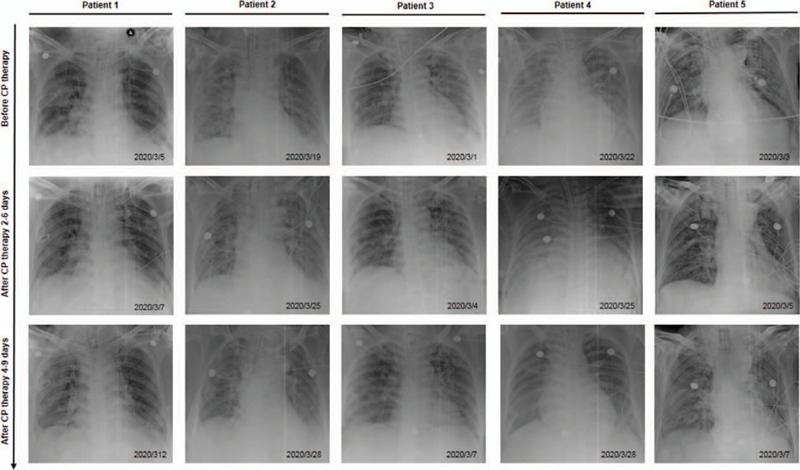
Changes in bedside chest radiographs in 5 critically ill COVID-19 patients before and after receiving the CPT. COVID-19 = coronavirus disease 2019, CPT = convalescent plasma therapy.
